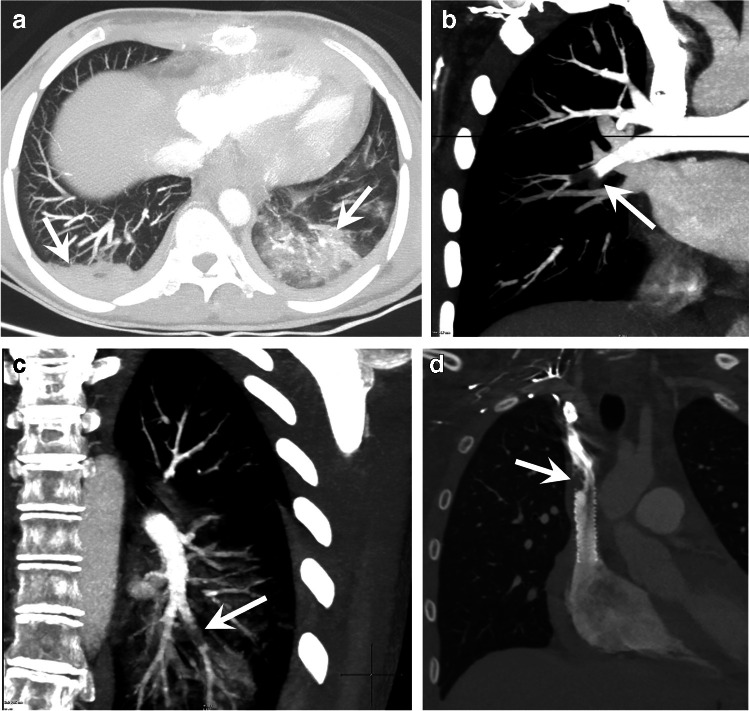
A 9-year-old girl with a heart transplant and a Dacron conduit in the superior vena cava (SVC). The patient presented with acute hypoxemia and pulmonary infiltrates. A contrast-enhanced computed tomography of the thorax was ordered to rule out pulmonary embolism. Bilateral pulmonary consolidations were seen on an axial maximum intensity projection (MIP) image (*arrows* in a). Angiographic coronal MIP images show occlusive thrombi in the pulmonary arteries of the lower lobes (*arrows*) in the right lung (b) and the left lung (c). A conduit-associated thrombus was seen on a higher window in coronal multiplanar reconstruction CT image (*arrow* in d)
